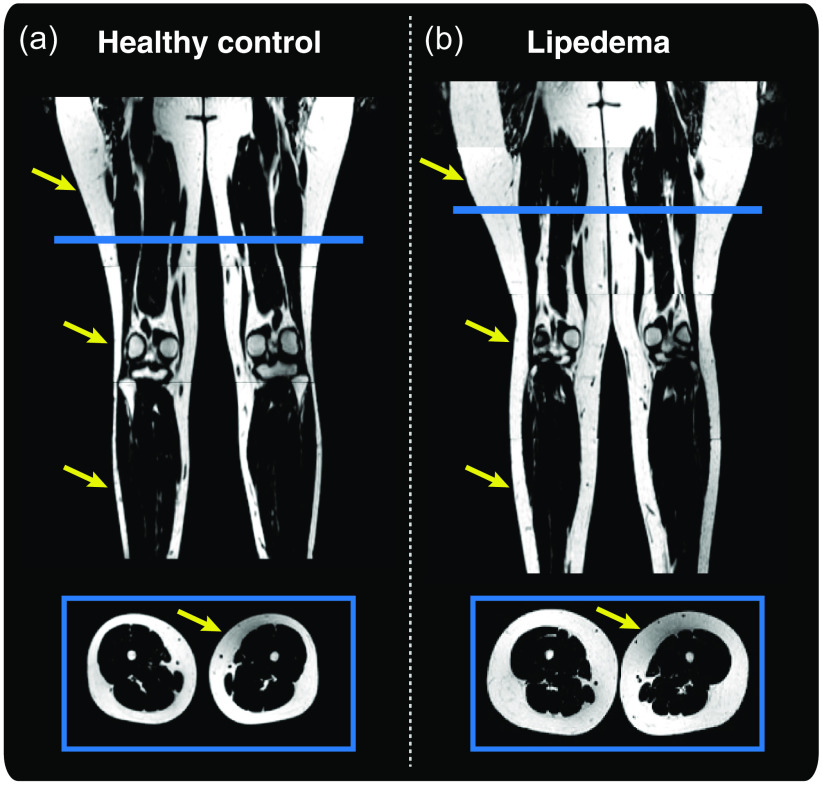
Representative example of (a) healthy female compared to a female patient with (b) lipedema. The healthy control is 28 years old with a BMI of $25.3\;\mathrm{kg}/m^{2}$ and the patient with lipedema is 23 years old with a BMI of $25.1\;\mathrm{kg}/m^{2}$. Structural measures of calf and thigh circumference are also similar (control versus lipedema values, calf circumference 37 cm versus 40 cm, thigh circumference 82 cm versus 86 cm). Lower extremity CSE-MRI reveals apparent thickened SAT in lipedema extending from thighs to ankles (yellow arrows). This is also observable on the transverse slice at the thigh level (blue box). SAT volume can be quantified by image analysis of musculoskeletal composition. In the midthigh region (decade 7): the mean SAT volume is greater for lipedema versus control 5.90 versus 3.89 mL, whereas mean muscle volume for lipedema versus control is 3.45 versus 4.16 mL. The SAT/muscle volume ratio in the midthigh is also greater in lipedema versus control 1.73 versus 0.94. Note: All stacks were rendered with an identical grayscale window/level, and image proportions were preserved.
